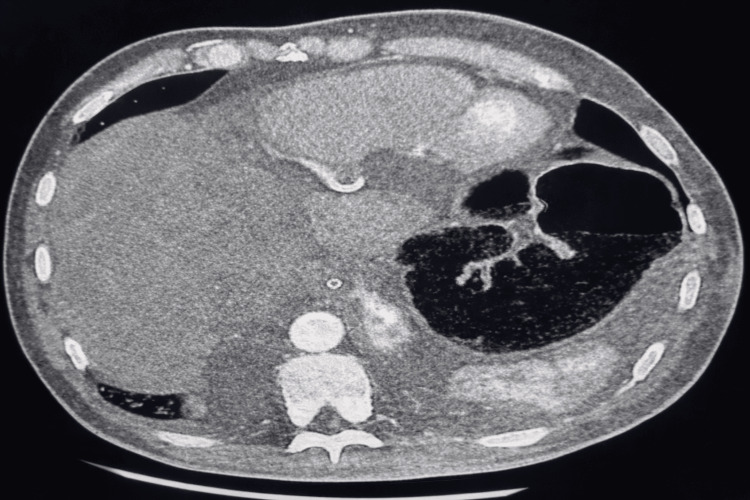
Abdominal contrast-enhanced CT scan Contrast-enhanced CT scan demonstrating a 9.2 cm hepatic abscess in segment VIII at the diaphragmatic dome.
